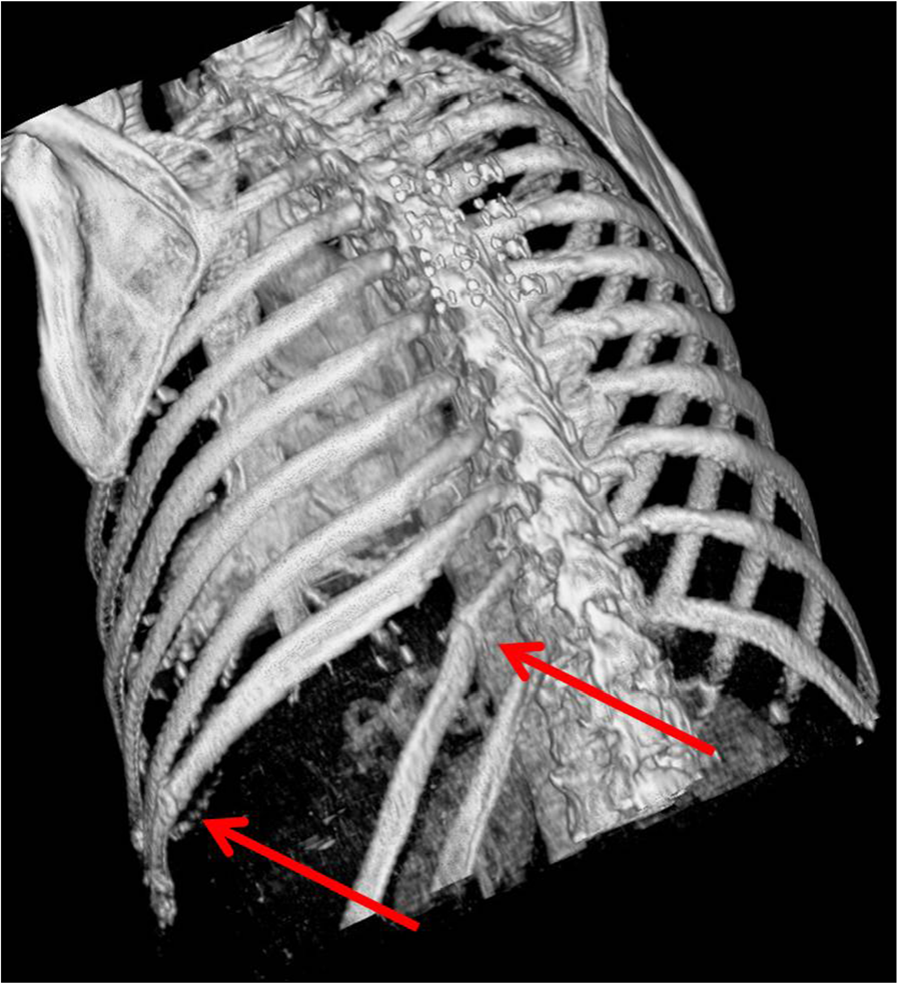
Three-dimensional reconstruction computed tomography image. The image shows left 10^th^ and 11^th^ rib fractures with splaying of the ribs secondary to underlying pseudohernia bulge (*arrows*)
